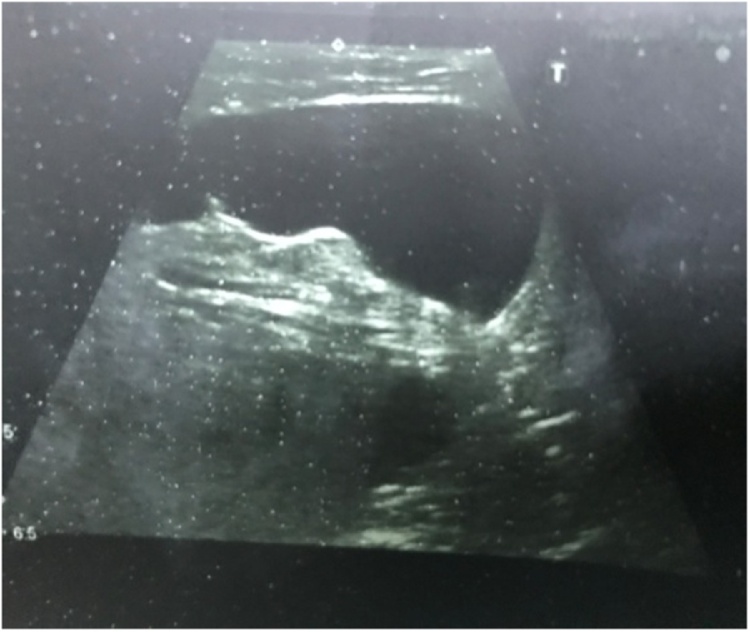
Cyst of the Canal of Nuck of Ultrasonography view.
